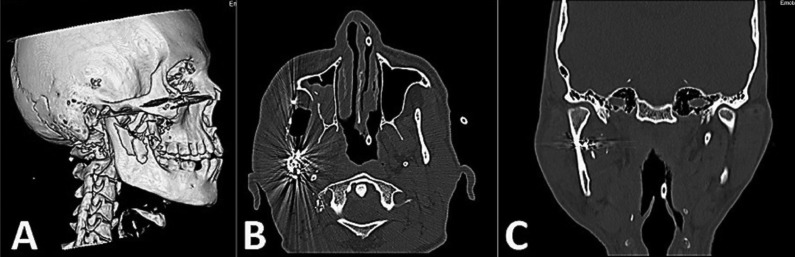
A three-dimensional projection of the CT scan. 2 e 3) CT of the facial bones demonstrates numerous fractures along the bullet trajectory.
